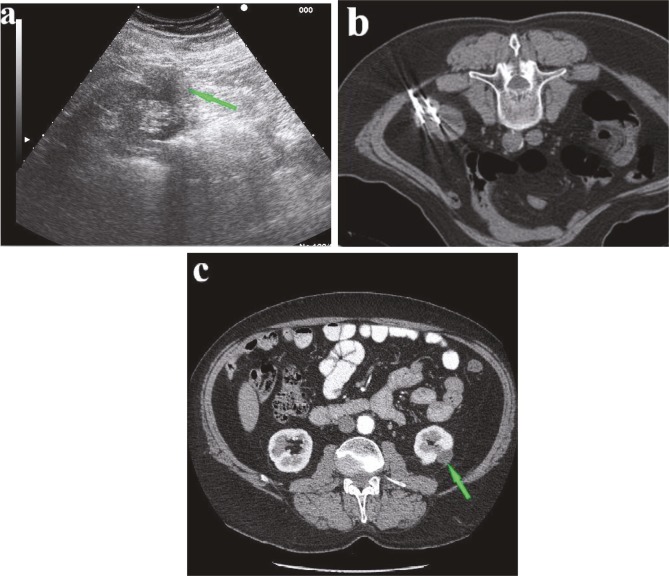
a) Seventy-year-old man with 4-cm biopsy-proven clear cell renal cell carcinoma (green arrow). RENAL nephrometry score calculated as 5. b) CT image obtained with patient in prone position shows cryoprobes in tumor. c) CT follow-up at 3 years demonstrates no evidence of tumor enhancement (green arrow).
